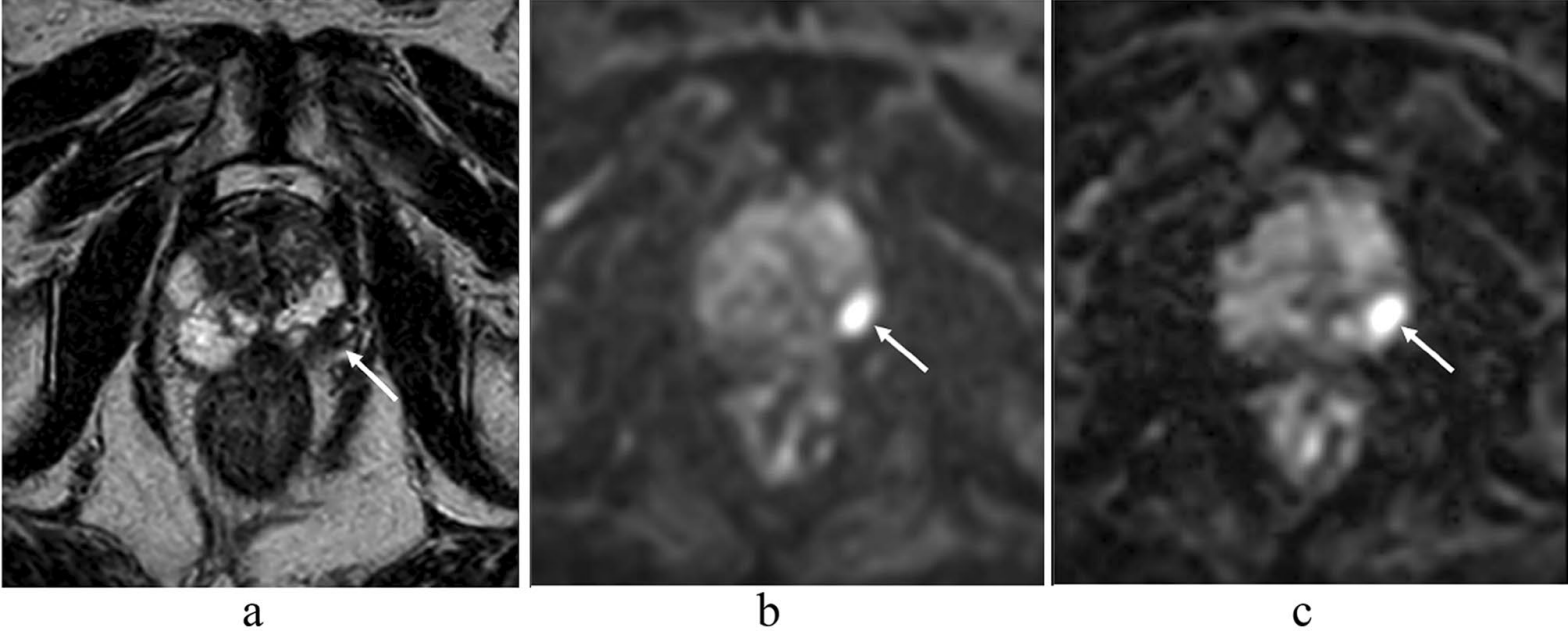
An 83-year-old man with prostate cancer (PSA level, 6.60 ng/mL; Gleason score, 3 + 4) in the left peripheral zone. A homogeneous hypointense lesion is seen on T2-weighted imaging (a) (arrow). A focal hyperintensity is depicted clearly on ssEPI DWI (b) and msEPI DWI (c) (arrow). The clarity of the prostate edge is better in msEPI DWI (c) compared with ssEPI DWI (b). *PSA* prostate-specific antigen, *ssEPI* single-shot echo-planar imaging, *DWI* diffusion-weighted imaging, *msEPI* multi-shot echo-planar imaging.
